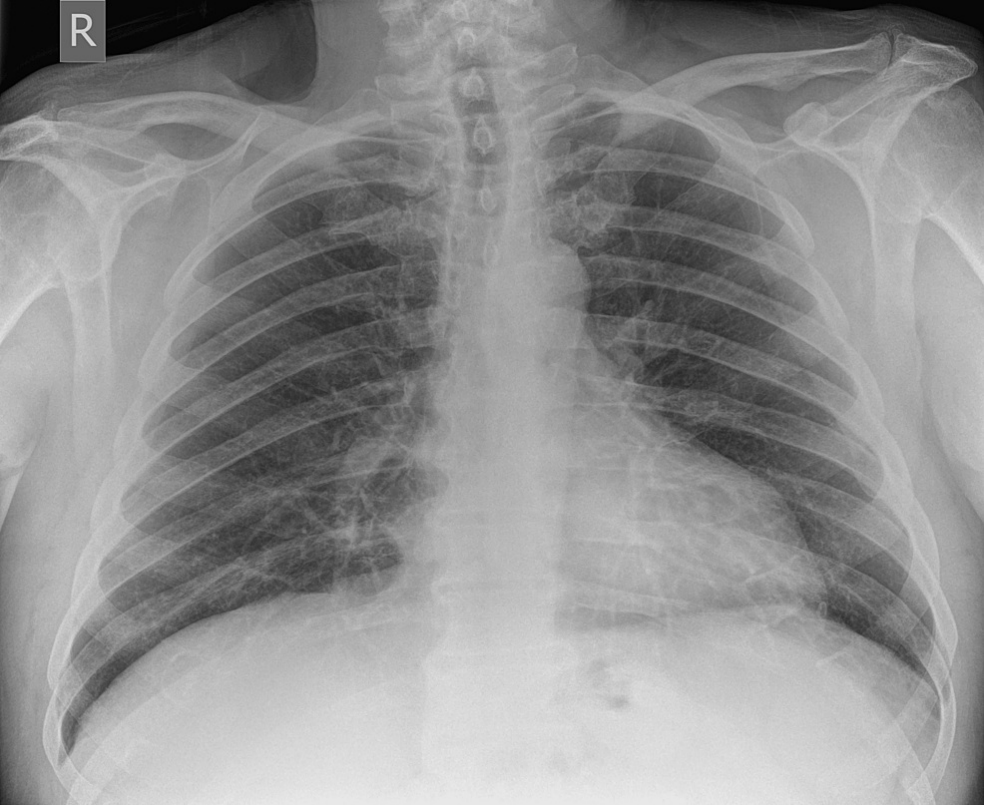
Preoperative chest X-ray Normal appearance on preoperative X-ray.
